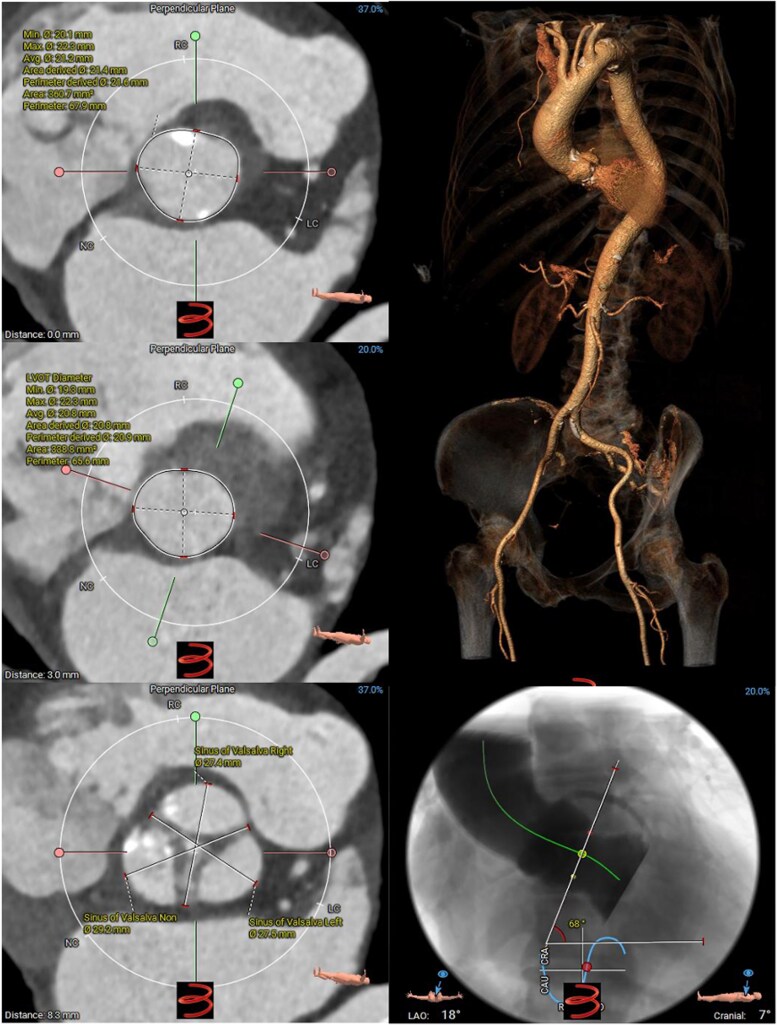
Case 2: CT TAVI for Case 2. Case planning identified the very horizontal aorta; however, a self-expanding system was preferred given the significant annular calcium.
